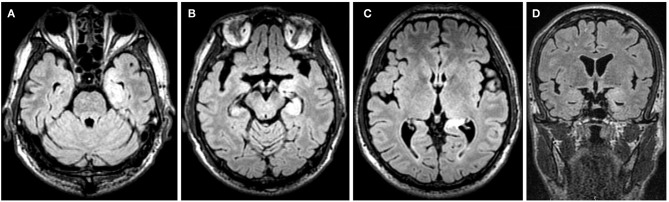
Case 17, male, 64 years old, who was diagnosed with anti-γ-aminobutyric acid B receptor (GABA_B_R) encephalitis. (A–D) Twenty-one days after onset and before immunotherapy, brain MRI showed high T2/fluid-attenuated inversion recovery (FLAIR) signal in the left hippocampus.
